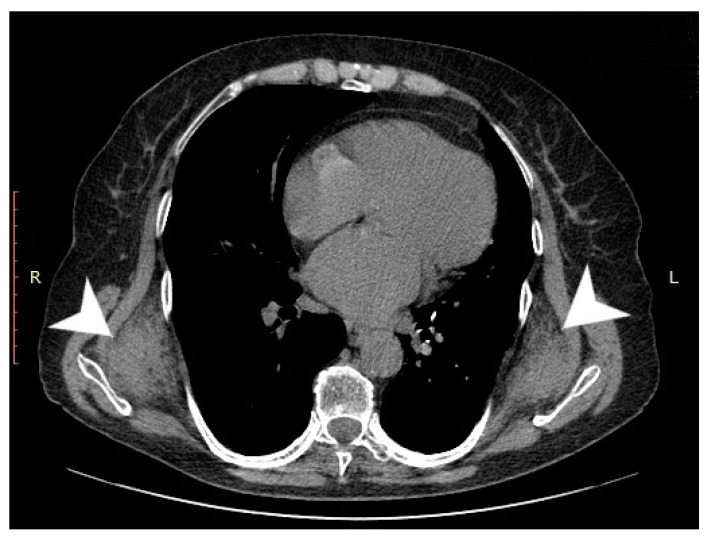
Axial CT view of the thoracic region at the level of the inferior third of the scapula. The tumoral masses are indicated by white arrows).
